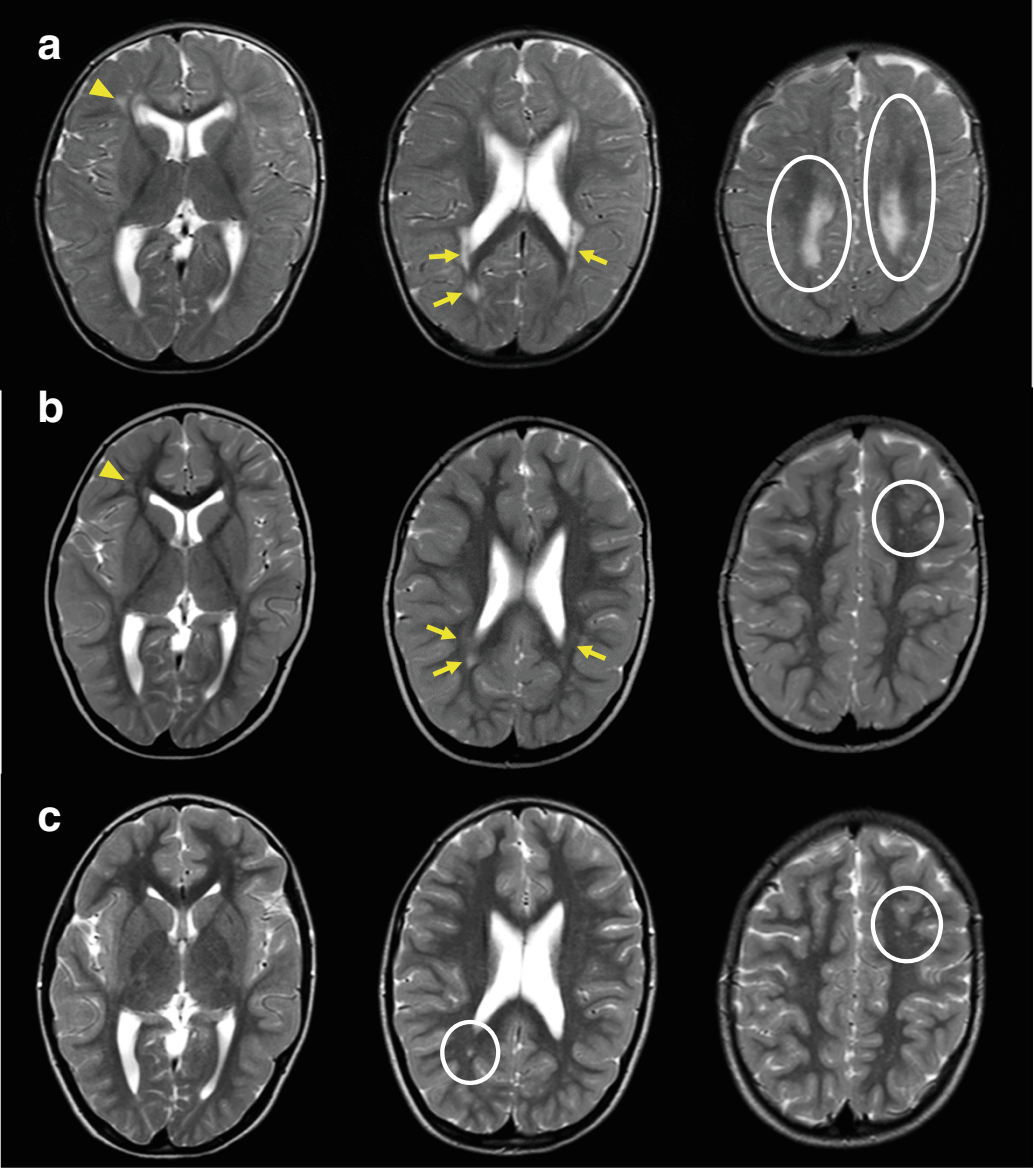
a Axial T2-weighted brain MRI. At 14 months of age (upper panels), abnormal areas of white matter were localized and remained around the lateral ventricle (arrow) and parietal lobes (round circles) in comparison to the findings at 1.5 months of age (see Fig. 1). The arrowhead indicates a periventricular cyst around the anterior horn of the right lateral ventricle. b At 3 years of age (middle panels), the abnormal white matter areas on T2-weighted images decreased further (arrow and circle). The periventricular cyst around the anterior horn of the right lateral ventricle was unchanged (arrowhead). c At 9 years of age (lower panels), a decreased volume of cerebral white matter was observed, but the abnormal areas of white matter had mostly disappeared, except for spotty signals in the parietal and occipital lobes (circles)
